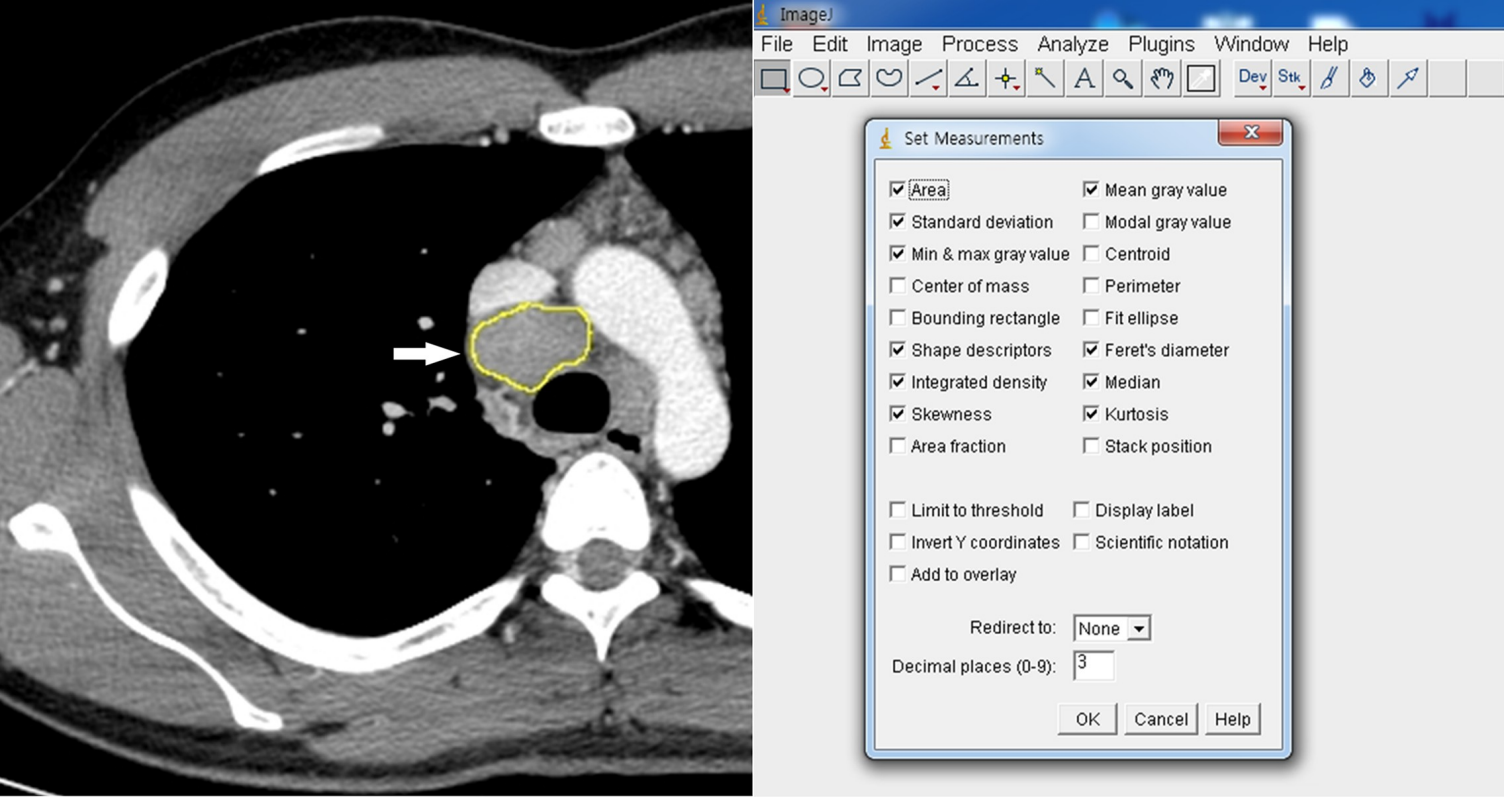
2D CT segmentation of the region of interest and quantitative CT analysis. (A) The lymph node of interest (arrow) was manually segmented on the image processing and analysis software using a graphic touch pen. (B) The evaluated measurements were specified in the dialog box of the image processing and analysis software.
